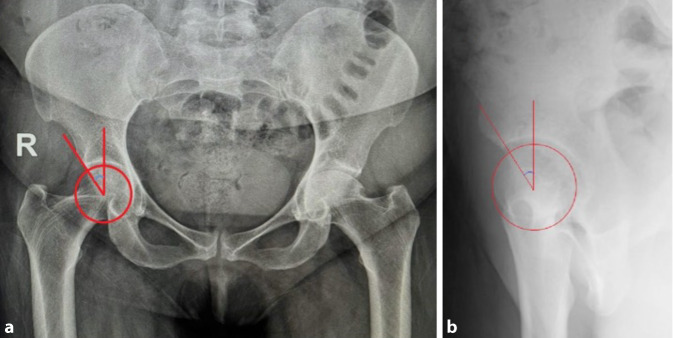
a AP pelvic radiograph shows LCEA on the right hip; The LCEA is the angle between the vertical line from the femoral head center and the line connecting the lateral margin of the acetabulum. b The right hip false profile radiograph shows ACEA; The ACEA is the angle between the vertical line from the center of the femoral head and the posterior margin of the acetabulum.
